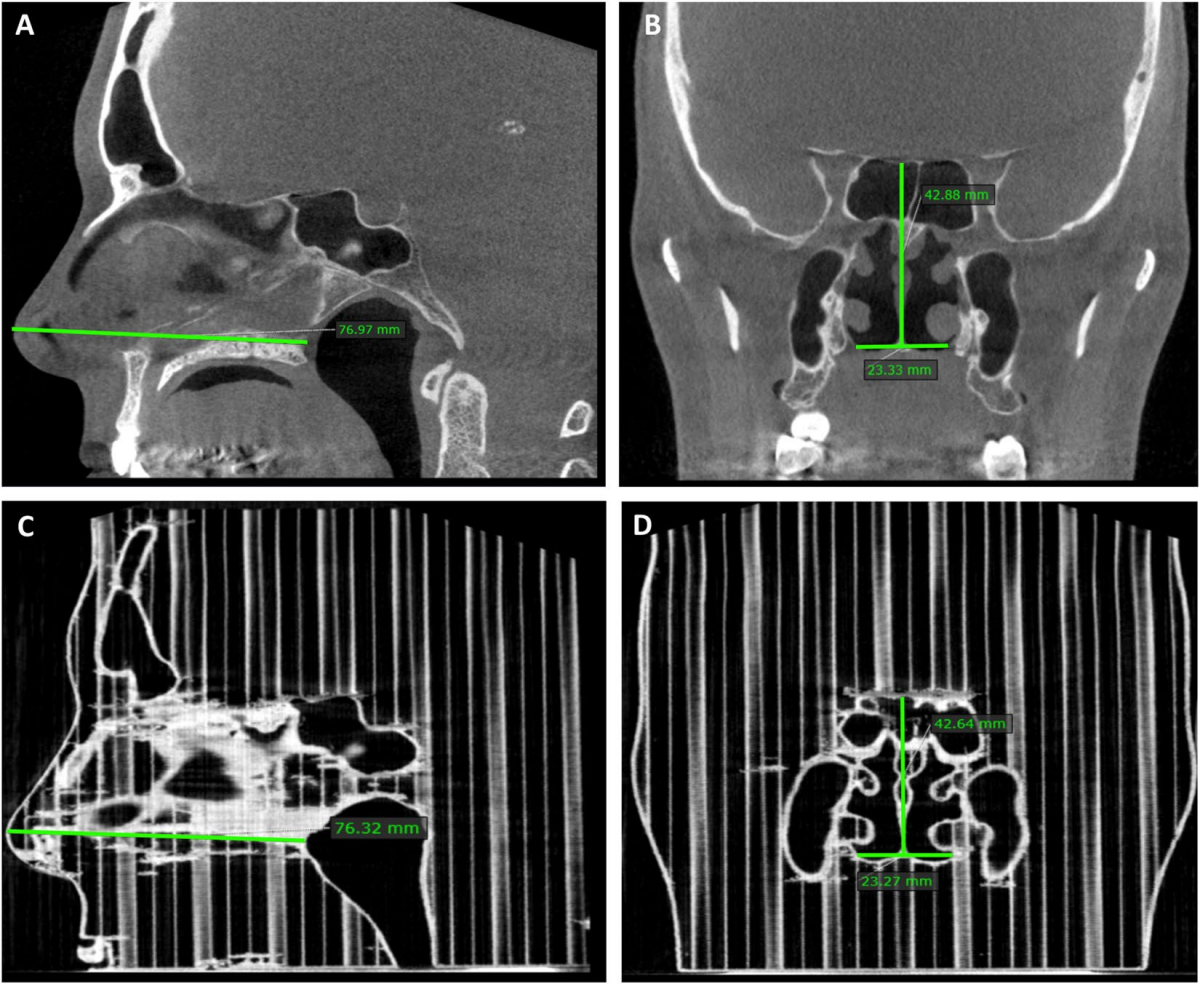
Linear measurements of the nose. Above: Septum length (A) and at the first endpoint of the nasal septum both nasal cavity height and width (B) in patient CBCT scans. Below: Similar measurements in the corresponding 3D print scans (C,D).
